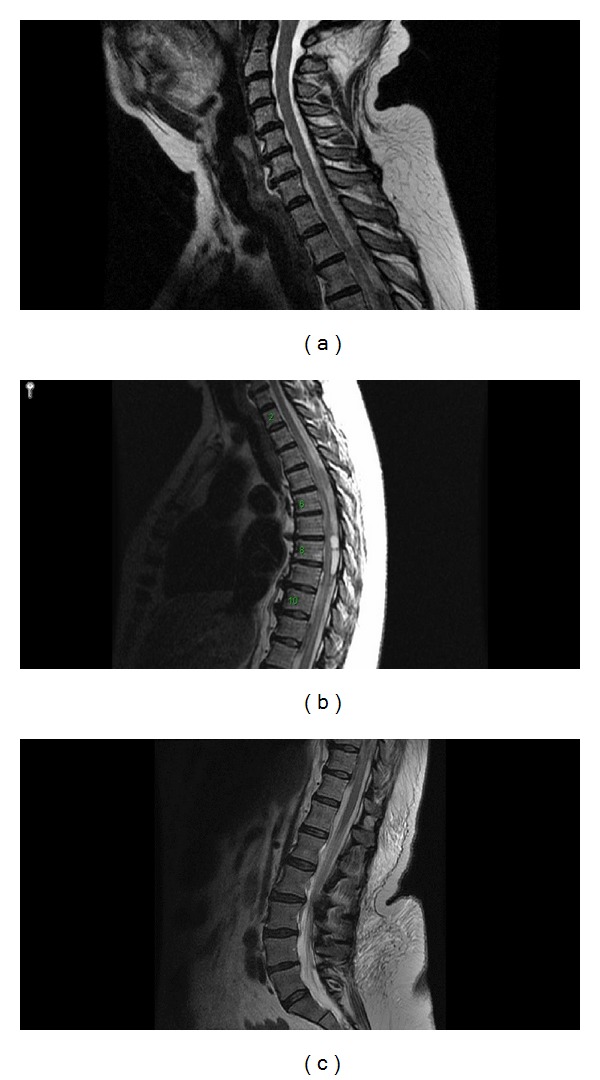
Sagittal T2 MR images of the cervical (a), thoracic (b), and lumbar (c) spine showing a complex cystic lesion in the dorsal spinal canal, spanning from above T1 to below T11, with spinal cord compression. Additionally, there is mildly increased T2 signal centrally within the spinal cord from the T3 to T11 level.
